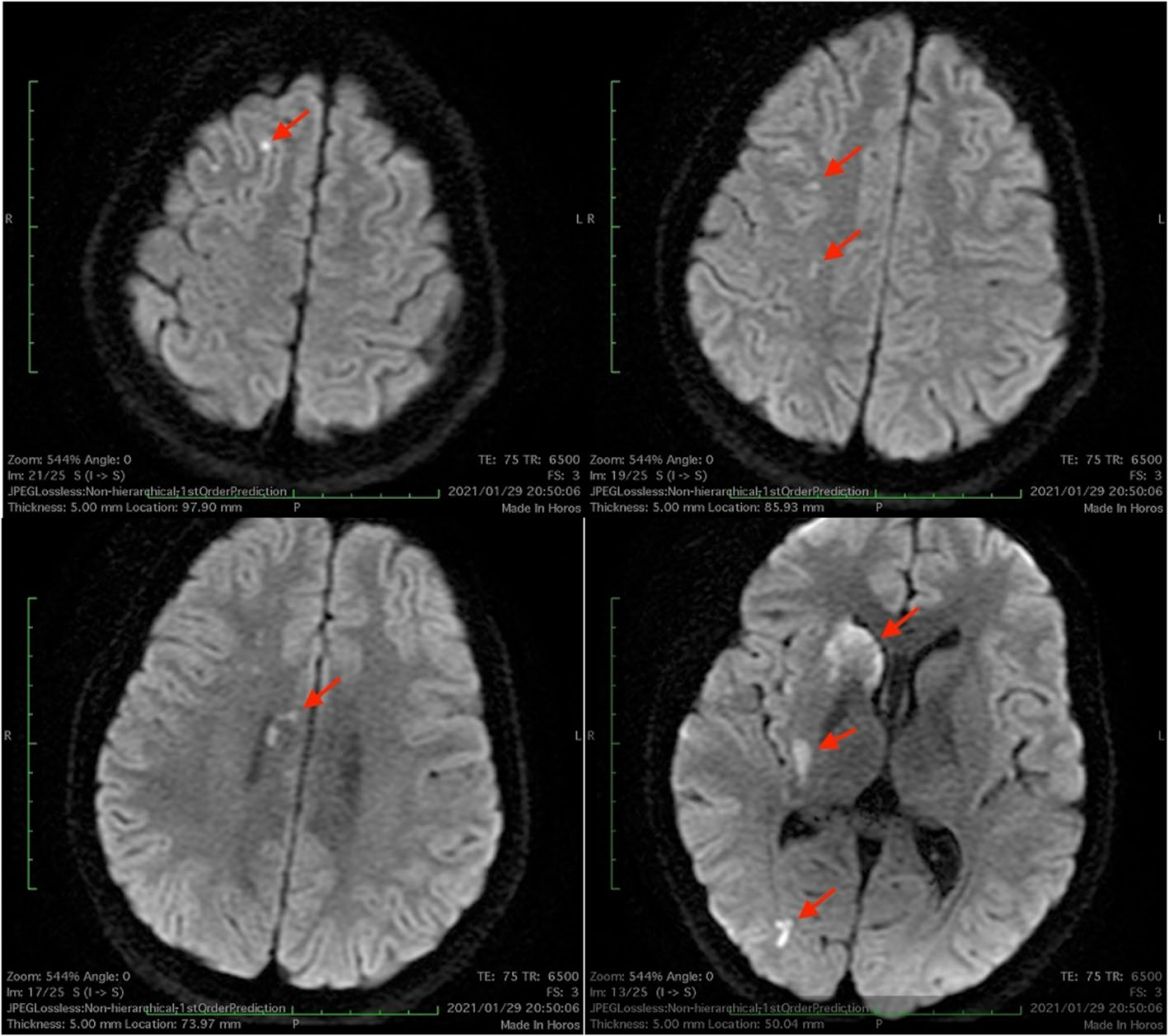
Brain magnetic resonance imaging reveals multiple areas of restricted diffusion (red arrows)
